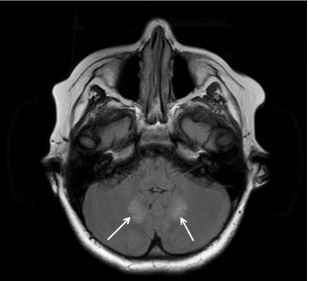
T2 and fluid-attenuation inversion recovery sequence magnetic resonance imaging of brain showing symmetric hyperintense dentate nuclei lesions that are characteristic of metronidazole-induced encephalopathy.
